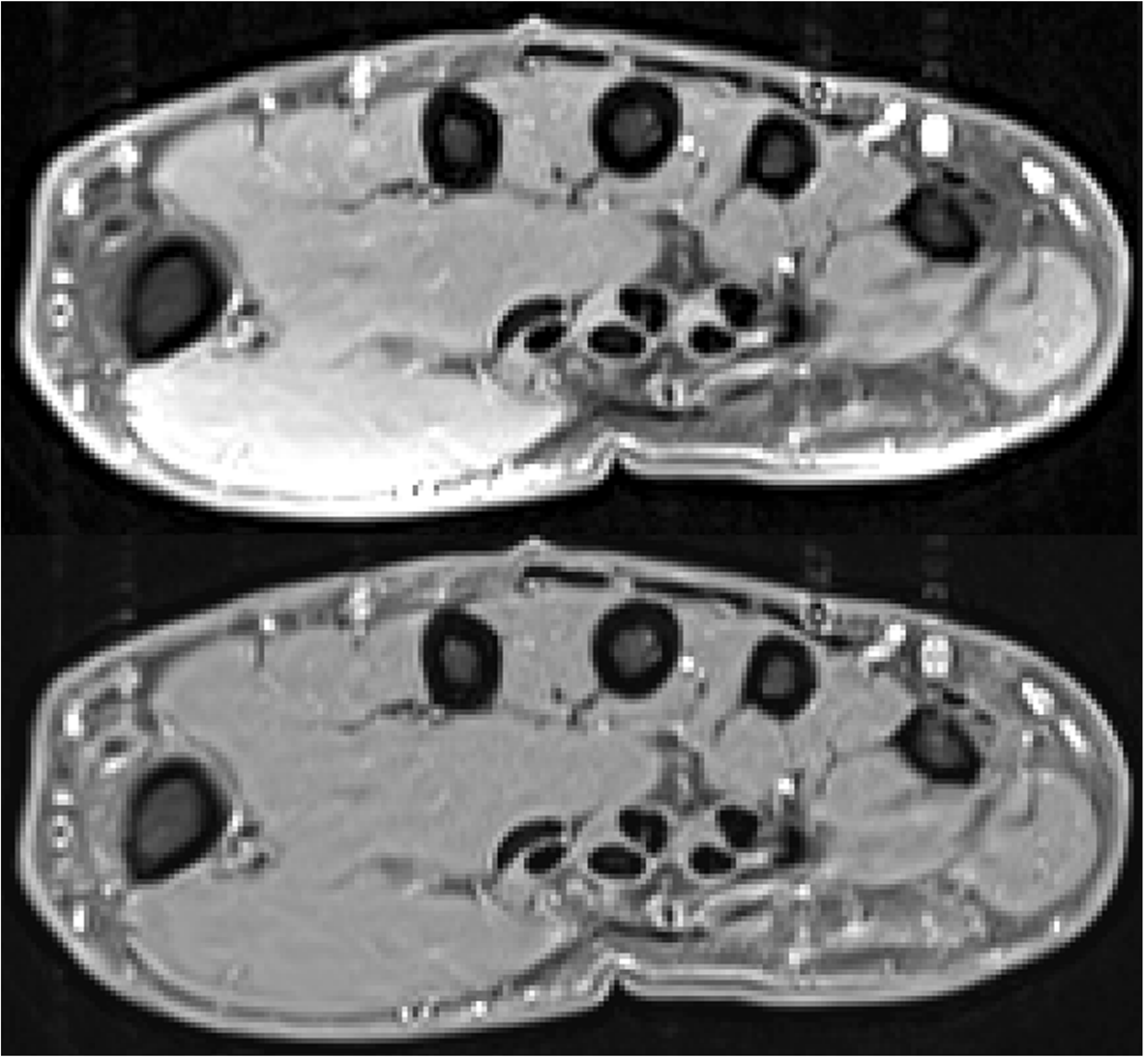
Top: Axial slice of a T1 weighted fat suppressed MRI hand scan in the metacarpal region. Notice the inhomogeneous intensity distribution of the grey values caused by a bias field, especially in the thenar region (lower left). Bottom: Same slice but with removed bias field by non-uniform intensity normalization (N4ITK)
